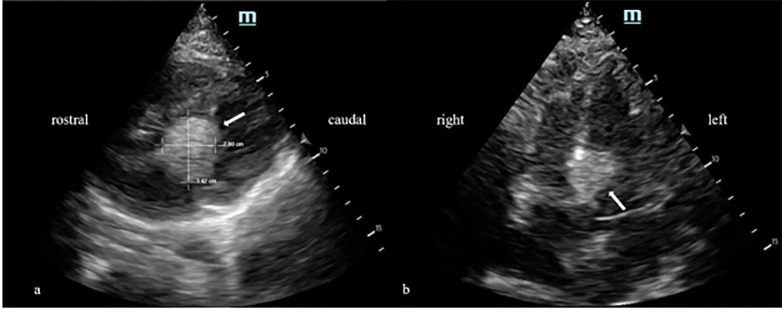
A) Parasternal long view demonstrating hyperechoic cardiac mass measuring 3.42 x 2.80 centimeters (arrow). B) Apical four-chamber view demonstrating same hyperechoic mass on septum (arrow).
